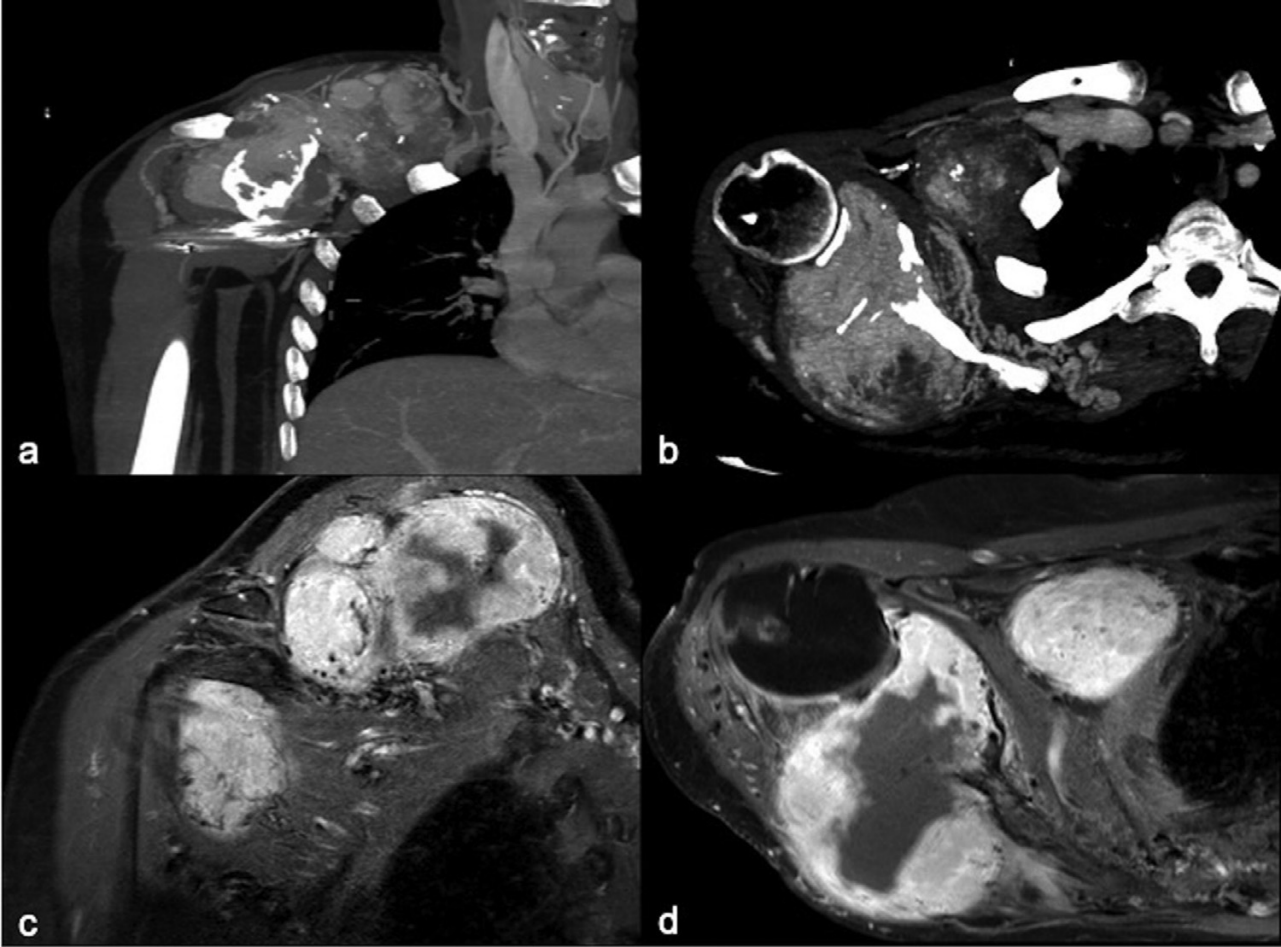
(A, B) Pretreatment Maximum Intensity Projection postcontrast venous coronal and axial CT before 2 combined treatment depicting the hypervascular hemangiopericytoma with erosion of the scapula. (C) Postcontrast coronal MRI after the 2 combined treatment focused in depicting the necrotic area in the medial portion of the lesion. (D) Postcontrast axial MRI after 2 combined treatment focused in depicting the necrotic area in the lateral portion of the lesion.
